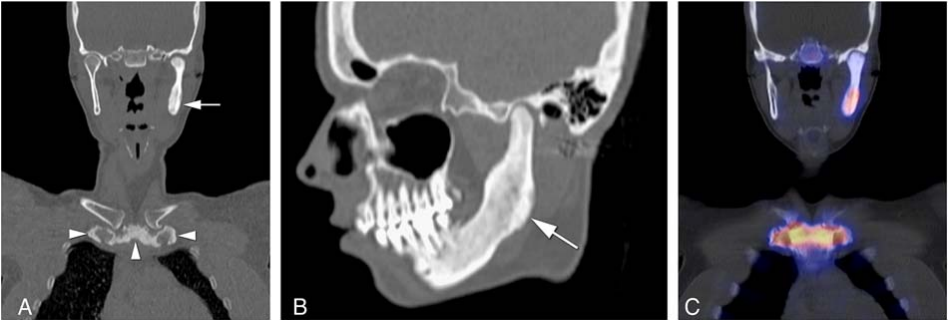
(A) Radiologic features of sclerosis and hyperostosis of the sternal manubrium and end of first ribs (arrowheads) and in the left mandible (arrow), on coronal reconstruction of computed tomography (CT) of the same patient as in Figure 2. Both clavicles are normal. (B) Sagittal reconstruction of CT of the same patient shows sclerosis and mild hyperostosis of the left mandible (arrow). (C) Increased uptake on SPECT-CT corresponding with increased osteoblastic activity (bone formation) is seen in the left mandible, sternal manubrium and medial ends of first ribs.
